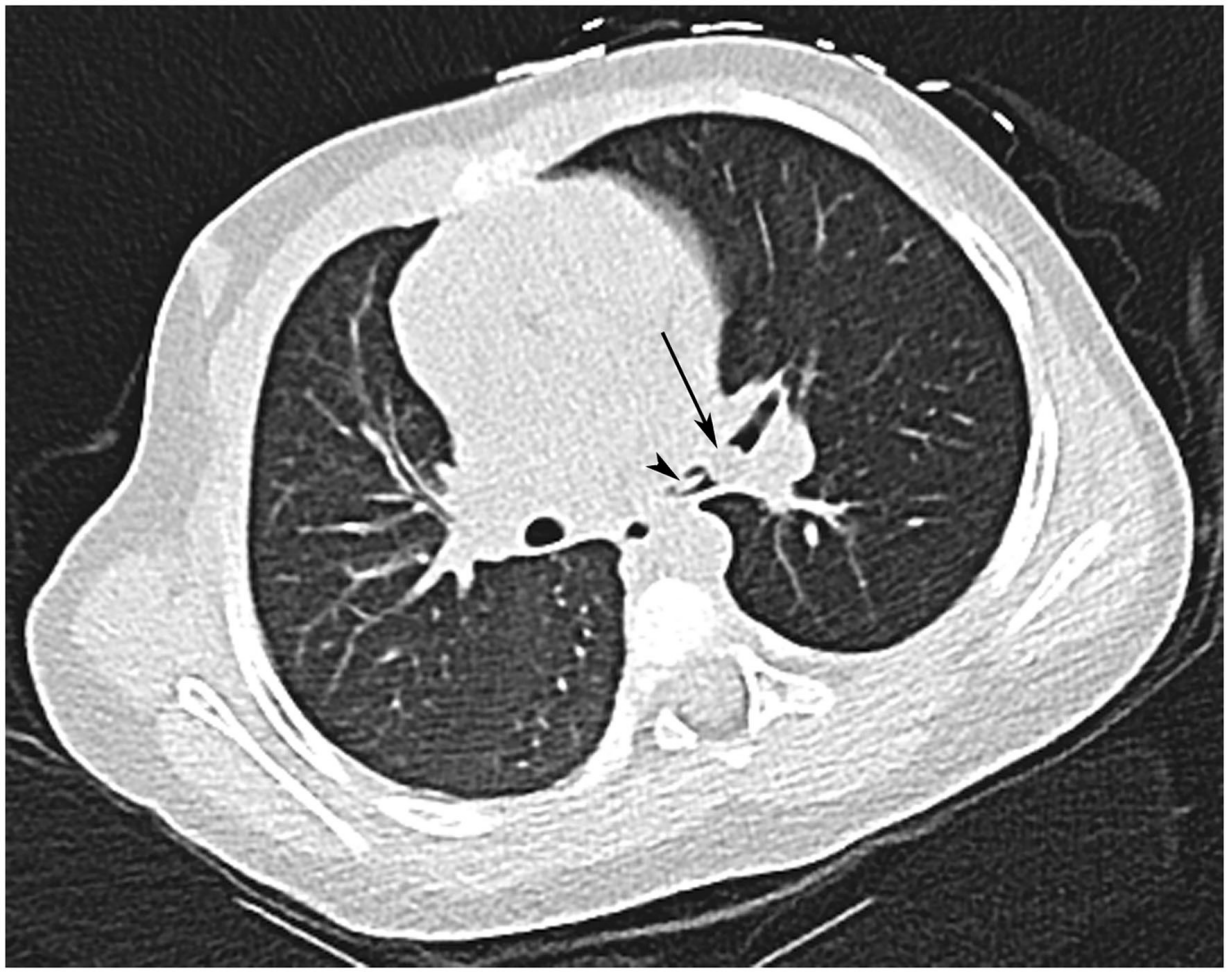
Inhaled foreign body in a 20-month-old female who choked on a piece of fruit. CT shows a fragment of fruit (arrow) and its stalk (arrowhead) at the bifurcation of the left main bronchus. Note also the decreased attenuation of the left lung.
